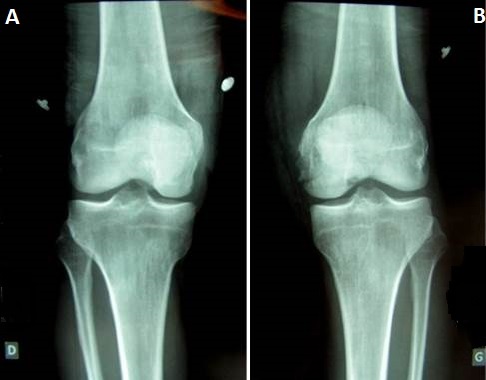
Antero-posterior radiographs of knees joint showing only of the left knee Hoffa fracture of the femoral medial condyle (A) right knee; (B) left knee
